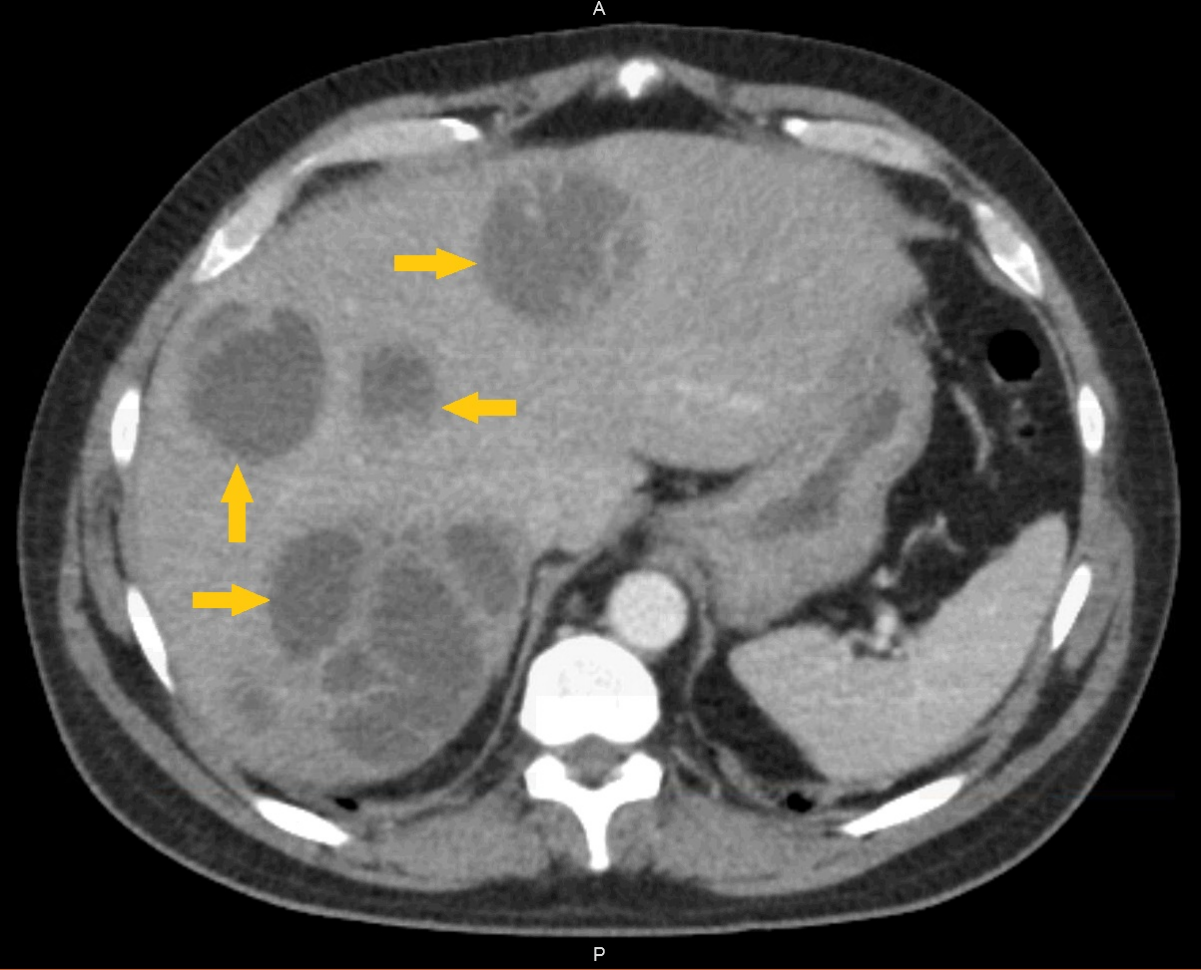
Initial CT scan of abdomen with arrows indicating multiple liver abscesses CT: computed tomography
